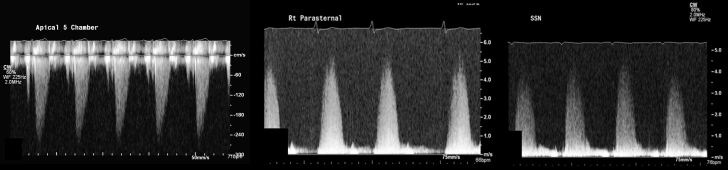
PEDOFF Probe Evaluation for Aortic Stenosis PEDOFF (nonimaging transducer) gradients obtained from the apical 5-chamber view, suprasternal notch, and right parasternal positions.
